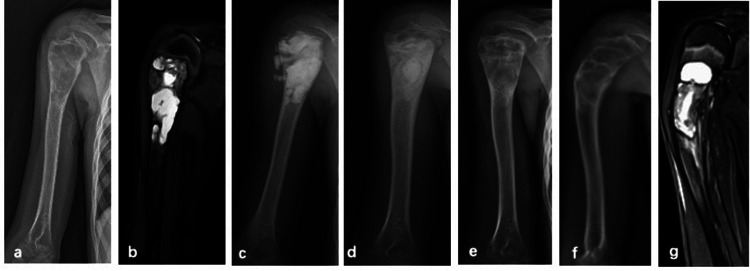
A 11-year-old male with SBC of left humerus treated by PAIB. (a,b) Radiograph of preoperative x-ray and MRI scan. (c) Immediate postoperative radiograph showed exudation of bone graft. (d) 3-month postoperative radiograph, the exudation of bone graft was absorbed. (e) 9-month postoperative radiograph showed recurrence was suspected. (f,g) Pathologic fracture occurred after operation 12 months by x-ray and MRI scan.
